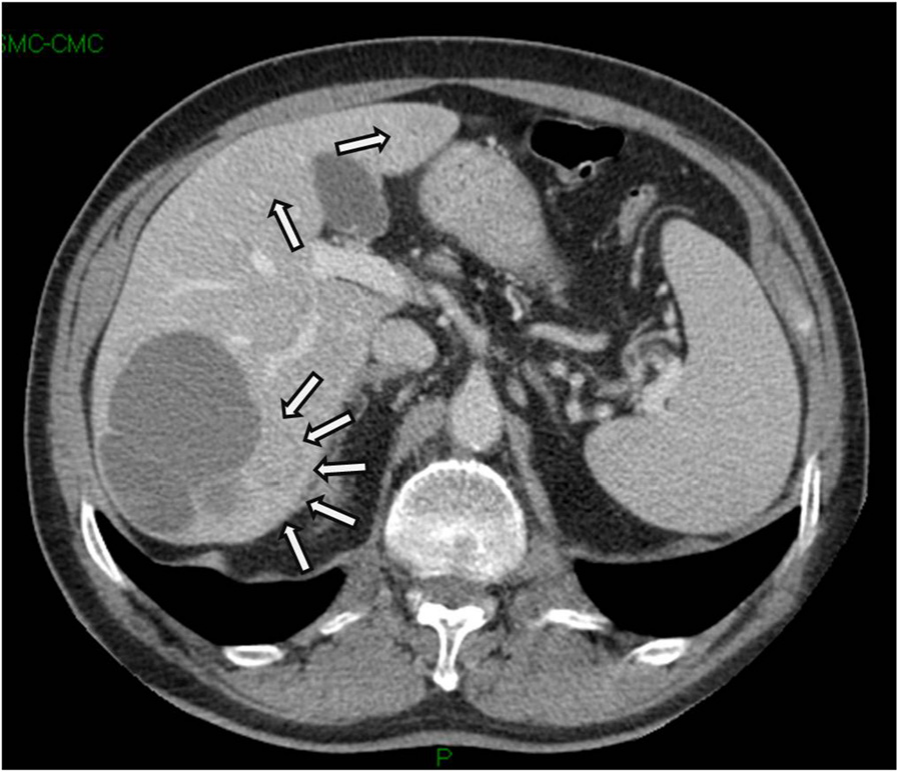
Computed tomography portal contrast phase image showing the presence of three slightly hypodense nodular lesions (arrows), the larger sited in the medial wall of a large cyst in segment VII.
